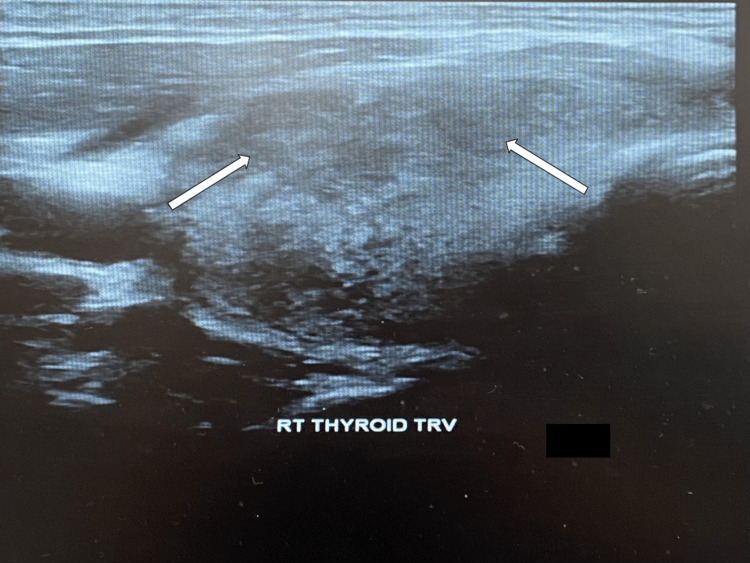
Ultrasound of enlarged heterogeneous thyroid with bilateral multiple nodules.
